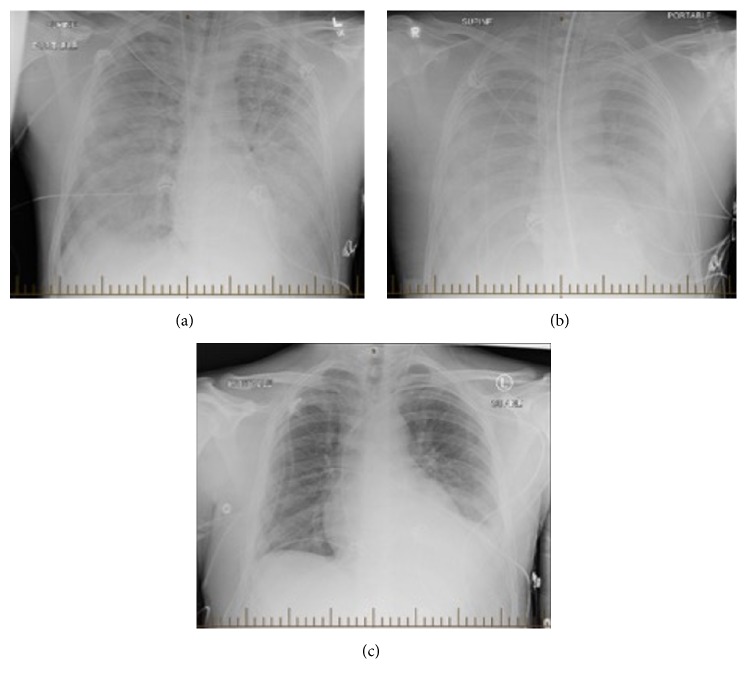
Radiographic progression of pulmonary disease in patient 1. (a) Chest radiograph on presentation; (b) chest radiograph on day two of admission following ECMO; (c) chest radiograph on day 10 of admission following extubation.
